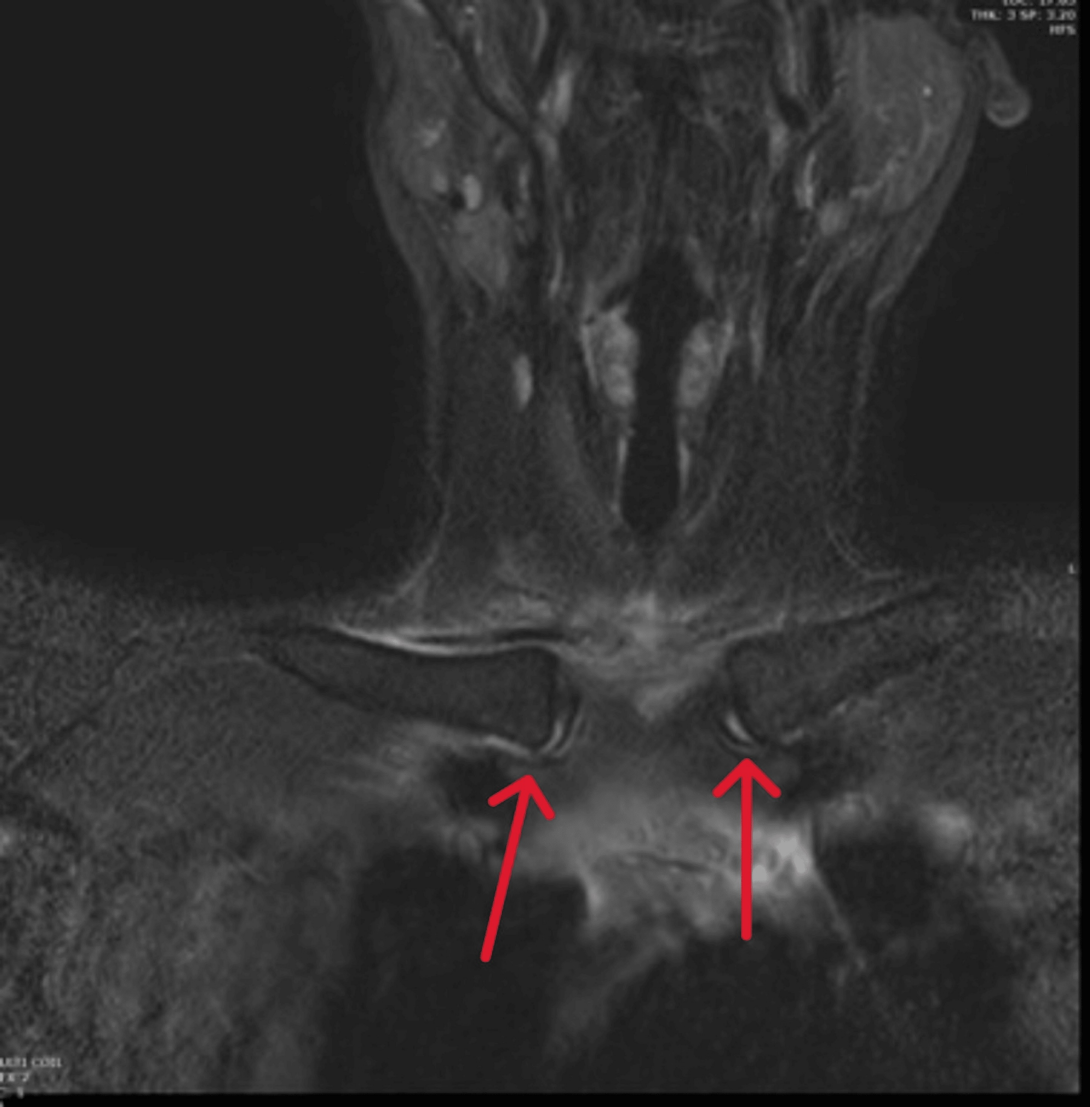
Coronal short tau inversion recovery (STIR) MRI revealed a fluid signal within both sternoclavicular joints (red arrows).
